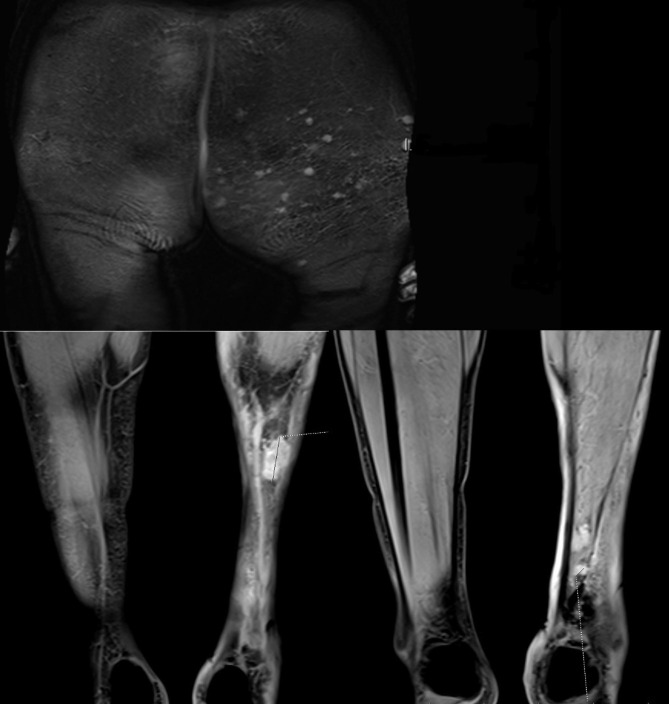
MRI of the lower extremities - multiple small subcutaneous nodules located in the left gluteal area, left calf and left hamstring area
